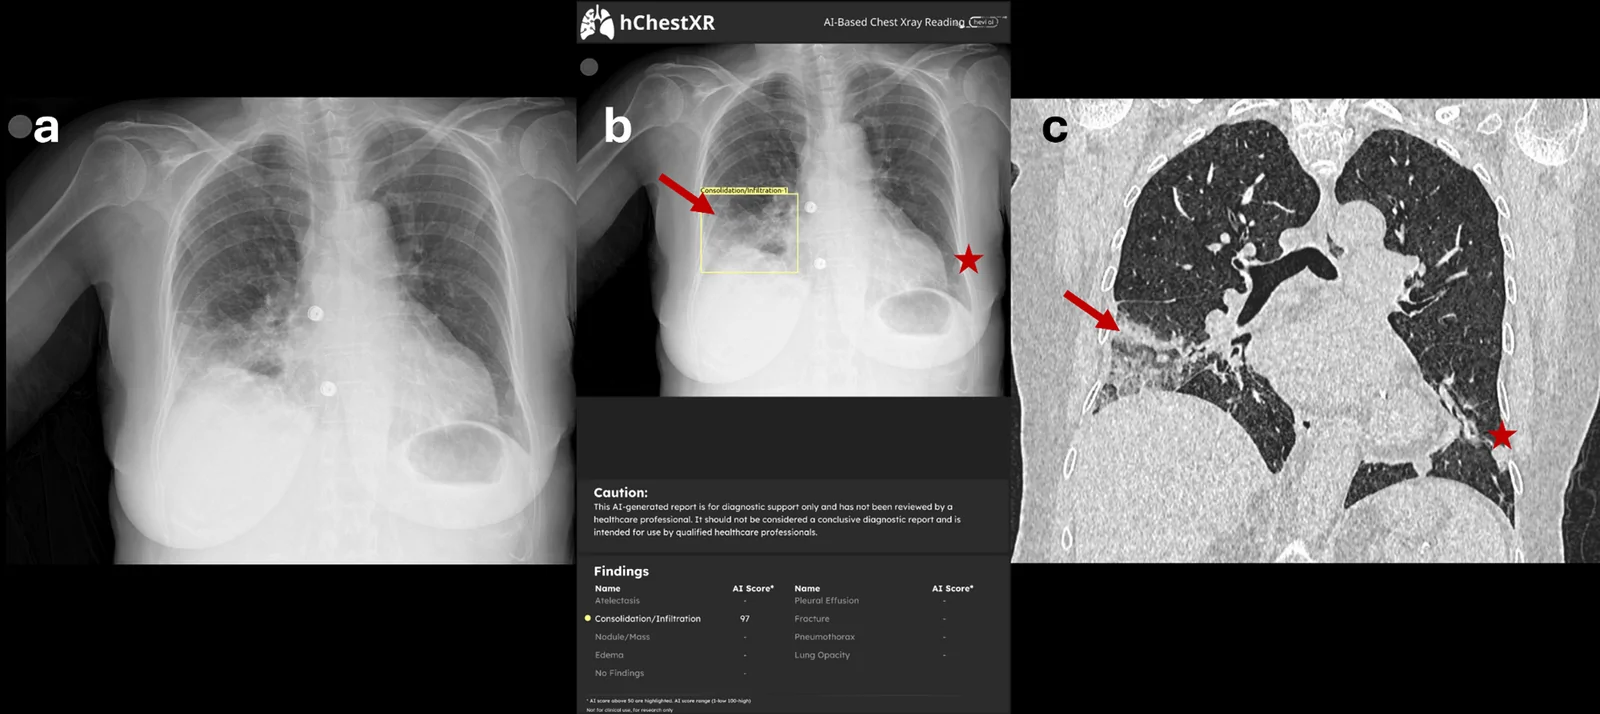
A 58-year-old female under surveillance for bladder cancer with a symptom of back and chest pain in Center C. (a) Posteroanterior chest radiograph of the patient. (b) The AI system identified the consolidation (arrow) in the right lower lung zone, yet it missed the visible nodule/mass in the left lower zone (star). (c) The corresponding coronal CT image demonstrates the consolidation in the right middle lobe (arrow) and solid subpleural lung nodule in the left lower lobe (star) missed by the AI system. This case was classified as true positive for the consolidation and false negative for the nodule.
